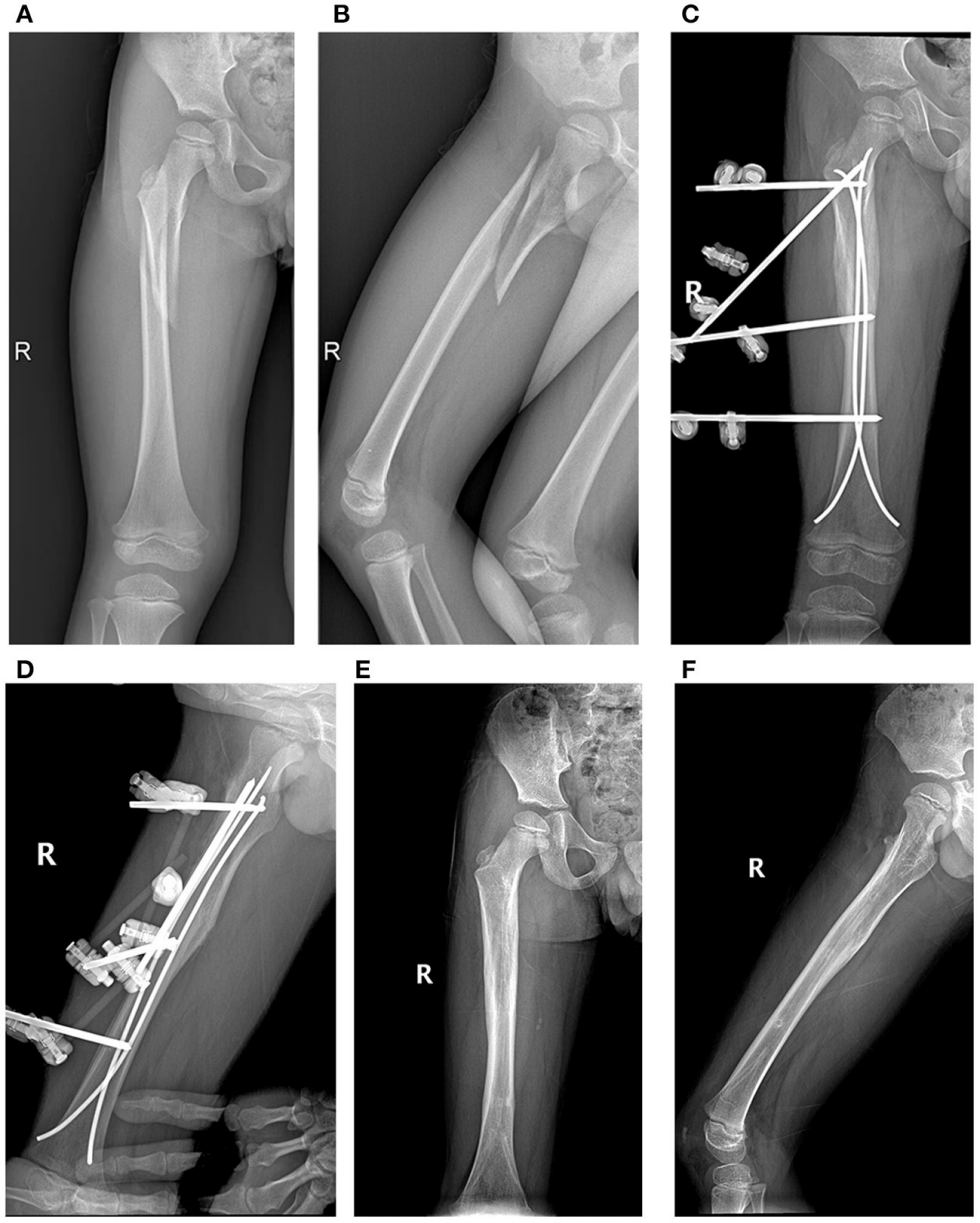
Case 18. (A,B) Long oblique proximal fracture of a 7-year-old boy; (C,D) postoperative radiographs; (E,F) final outcome.
